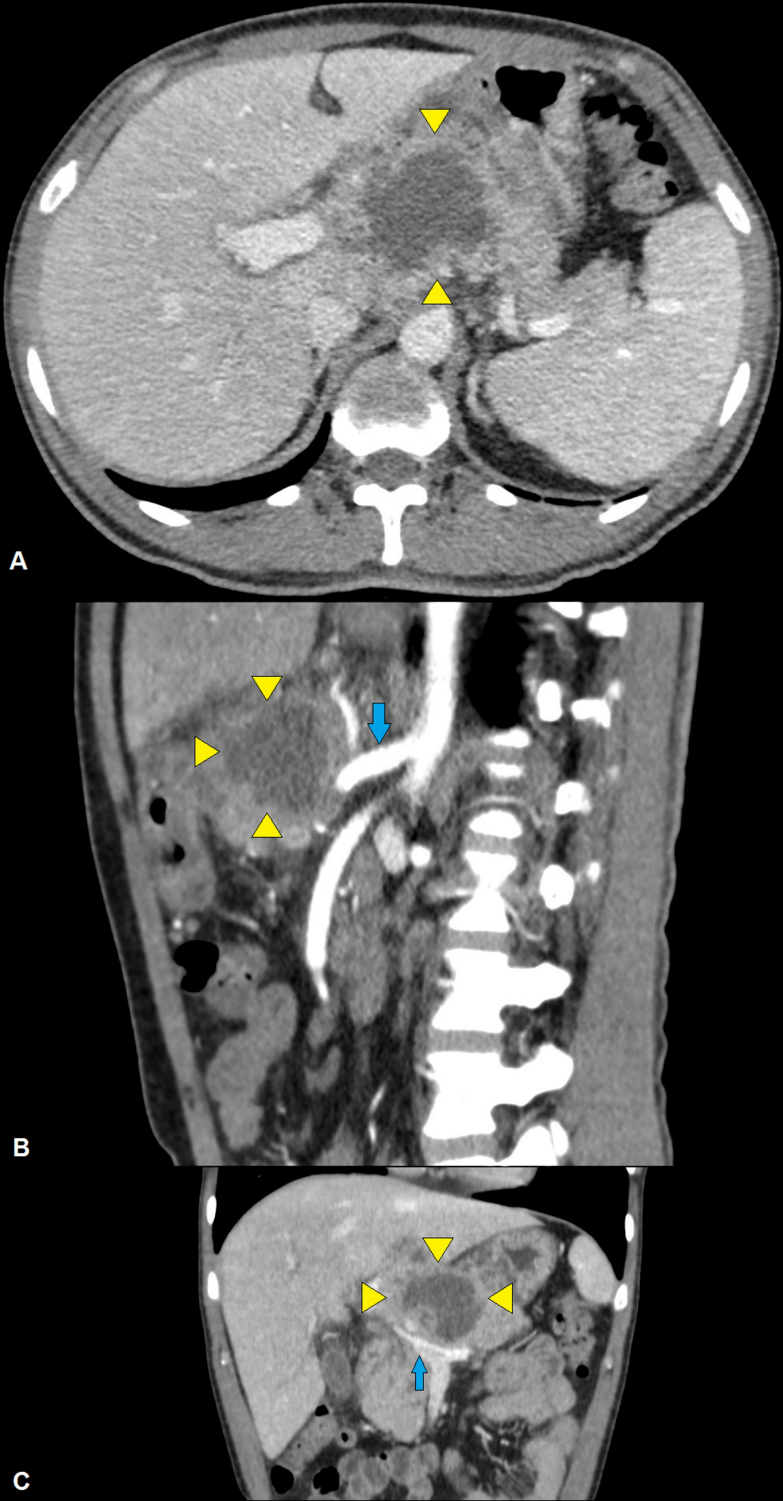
Computed tomography of the abdomen (A) A large heterogeneous necrotic mass (arrowheads) is seen arising from the pancreatic body with loss of fat planes and possible infiltration of the left lobe of the liver. (B) The mass (arrowheads) encases the celiac vessels (arrow) and (C) portal vein (arrow).
